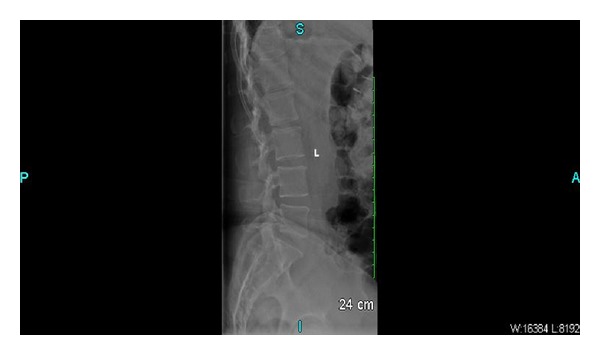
Plain lumbosacral X-ray showing degenerative changes.
